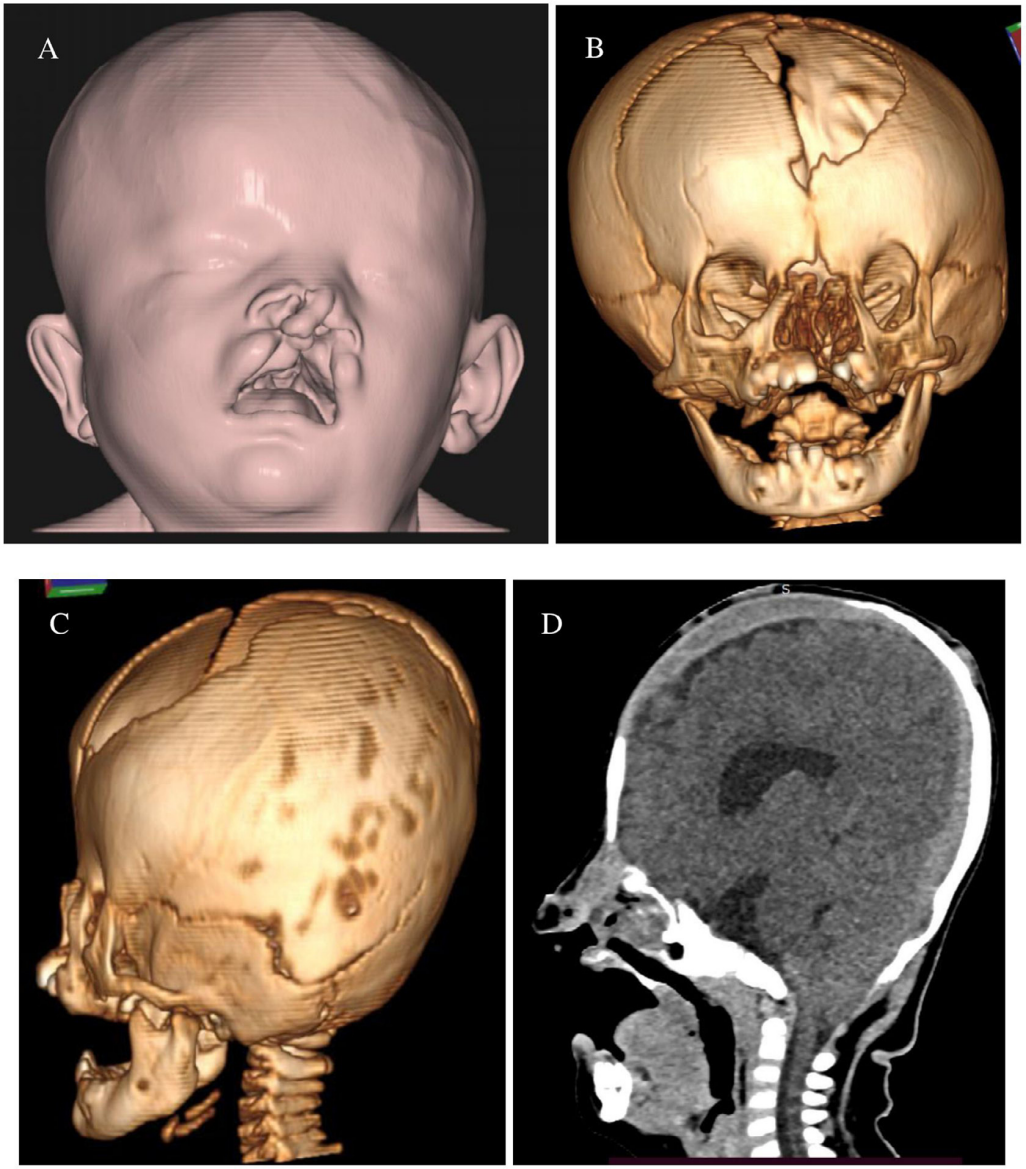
Head three-dimensional (3D) volume rendering (A) and head bone 3D reconstruction (B) showed defects in the nasal bone, right and left medial orbital wall, and cleft lip and palate. The 3D reconstruction of the left side (C) showed a craniosynostosis at the left lambdoid suture, left coronaria suture, and frontalis suture. Sagittal head computed tomography (CT) scan of the cranial window (D) revealed hypogenesis of the corpus callosum and tonsillar cerebellar herniation.
